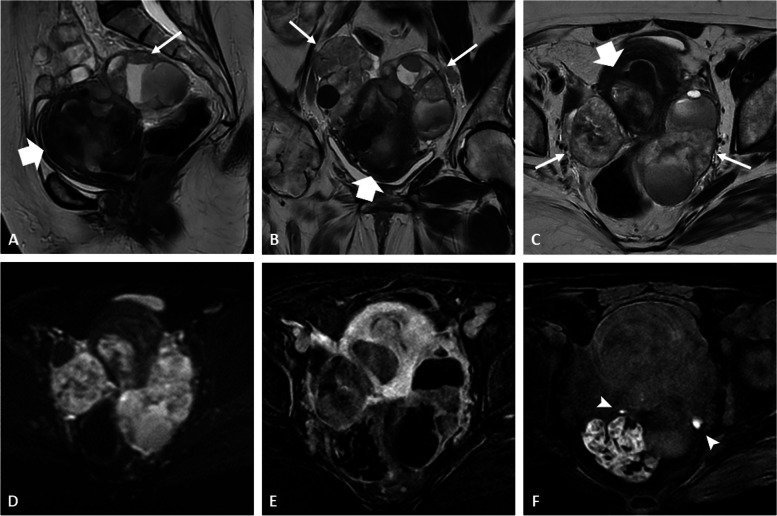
Differential diagnosis: endometrial carcinoma with bilateral ovarian metastases in a 57-year-old woman with endometriosis. T2-weighted images in the sagittal (a), coronal (b), and axial (c) plane show a large intra-uterine mass (thick arrows) and bilateral ovarian masses (thin arrows). All lesions display restricted diffusion (d high *b* value) and enhancement on axial contrast-enhanced fat-suppressed T1-weighted images (e). Small-sized hemorrhagic foci within the ovarian masses are shown on fat-suppressed T1 weighted image (arrowhead in f), corresponding to superficial endometriosis lesions on the ovarian surface. Surgery confirmed a high-grade endometrial carcinoma with ovarian metastases and underlying endometriosis
